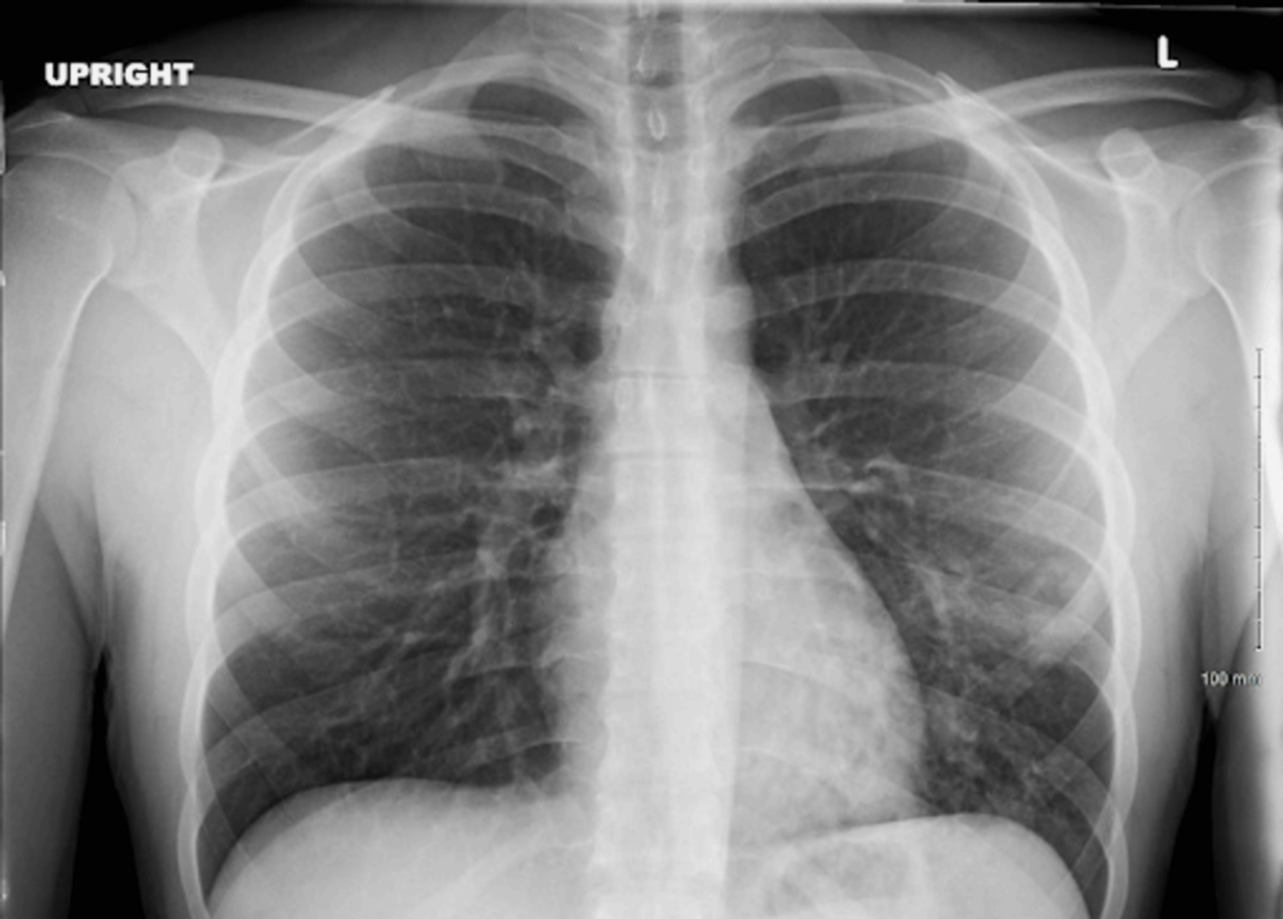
An upright anterior-posterior chest radiograph showing no remarkable abnormalities, such as infiltrates, consolidations, cavities, or lymphadenopathy associated with a pulmonary tuberculosis infection.
